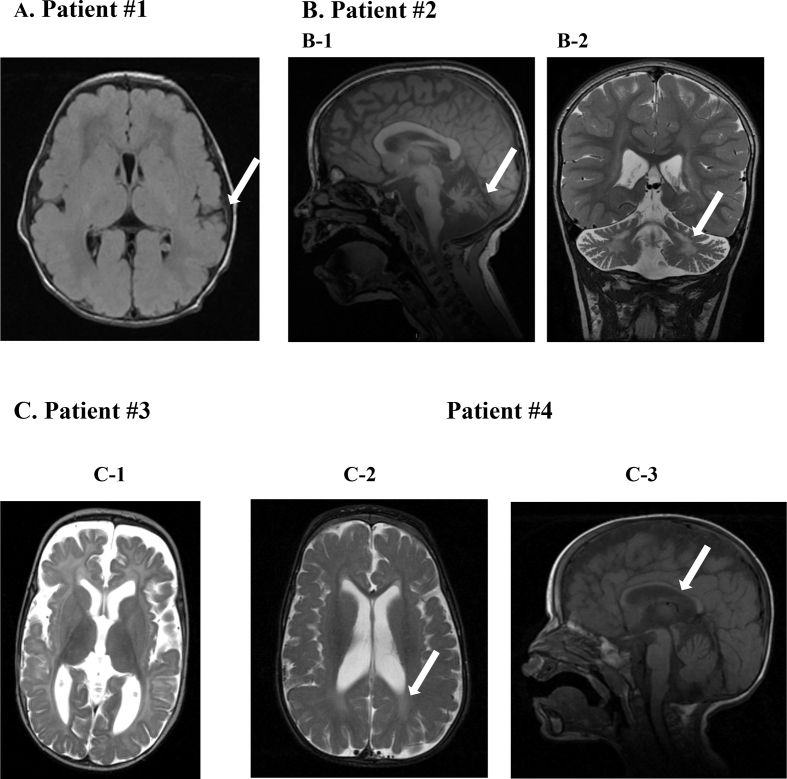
Brain MRI of the patients. A. An axial T2 Flair sequence of patient #1 at 6 days of age demonstrating mild bilateral peri-sylvian polymicrogyria (arrow). B. Patient #2 - MRI performed at 5 years. Significant cerebellar atrophy and mild cerebral atrophy are noted on the sagittal T1 view (B-1) and abnormal signal in the dentate and cerebral WM are demonstrated in the coronal T2 view (B-2) (arrows). C. Patients #3–4. C1) An axial T2 sequence of patient #3 at 8 months of age - delayed white matter maturation, enlarged ventricles, and extra-axial fluid; C-2) An axial T2 sequence of patient #4 at 18 months of age demonstrating abnormal periventricular white matter signal (arrow); C-3) A sagittal T1 sequence of patient #4 at 18 months of age showing thin corpus callosum (arrow), mild cerebellar atrophy, and extra-axial fluid.
